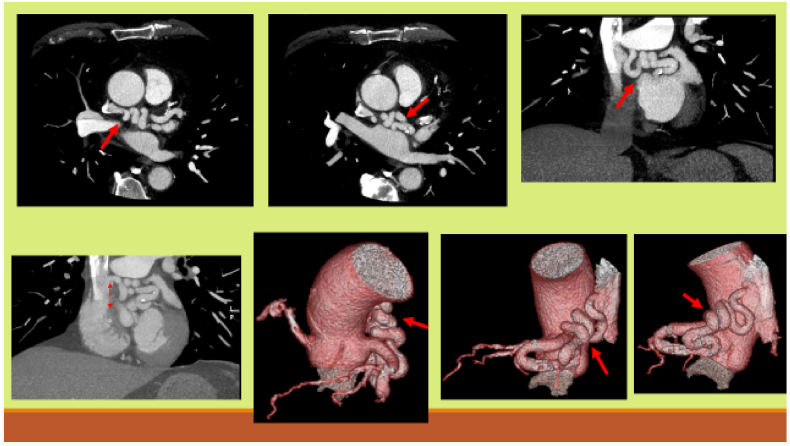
Several CT sections of the fistula.
